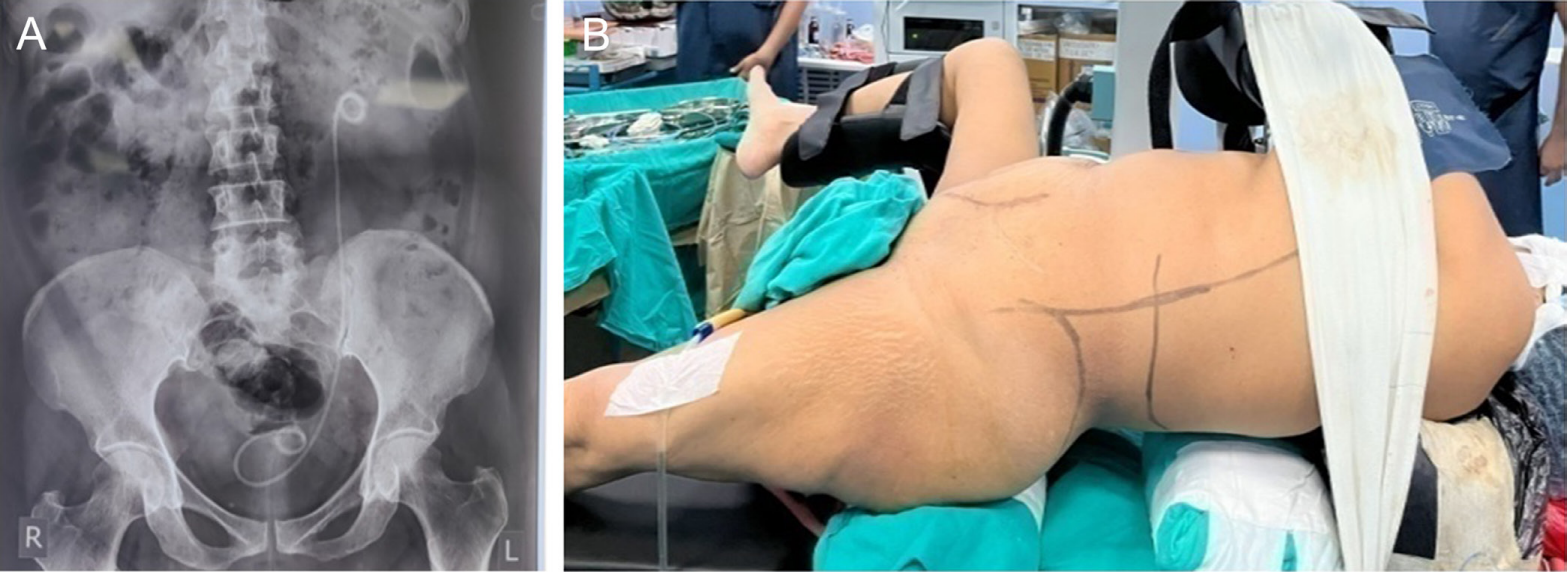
(A) Preoperative x-ray showing left encrusted DJ stent with renal calculus as well as bladder calculus in an obese patient. (B) Calcutta-modified supine position with markings.
